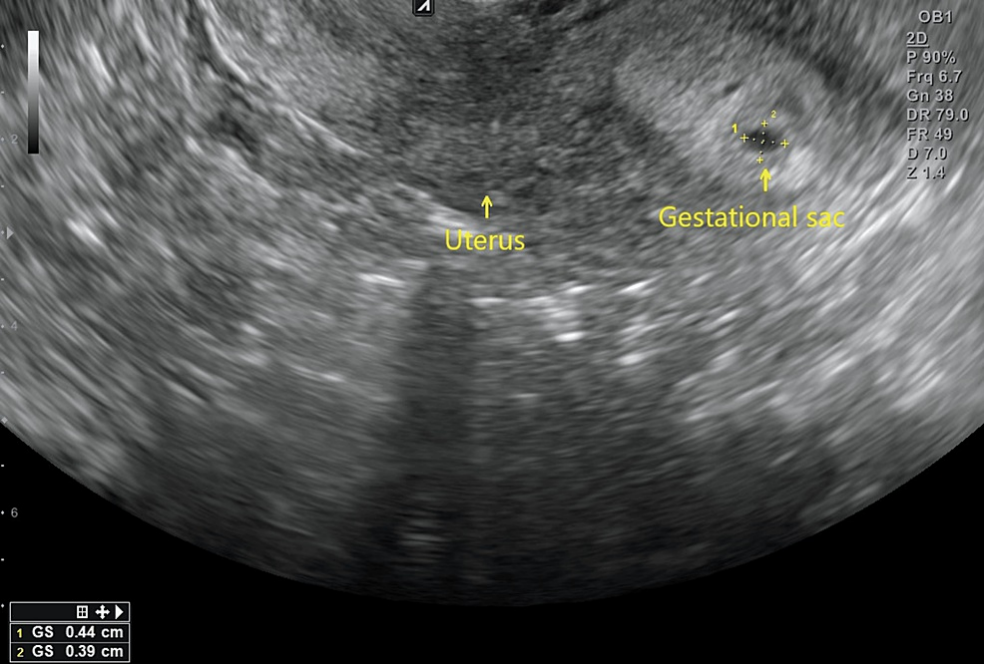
Ultrasonographic confirmation of pregnancy via locating the gestational sac
